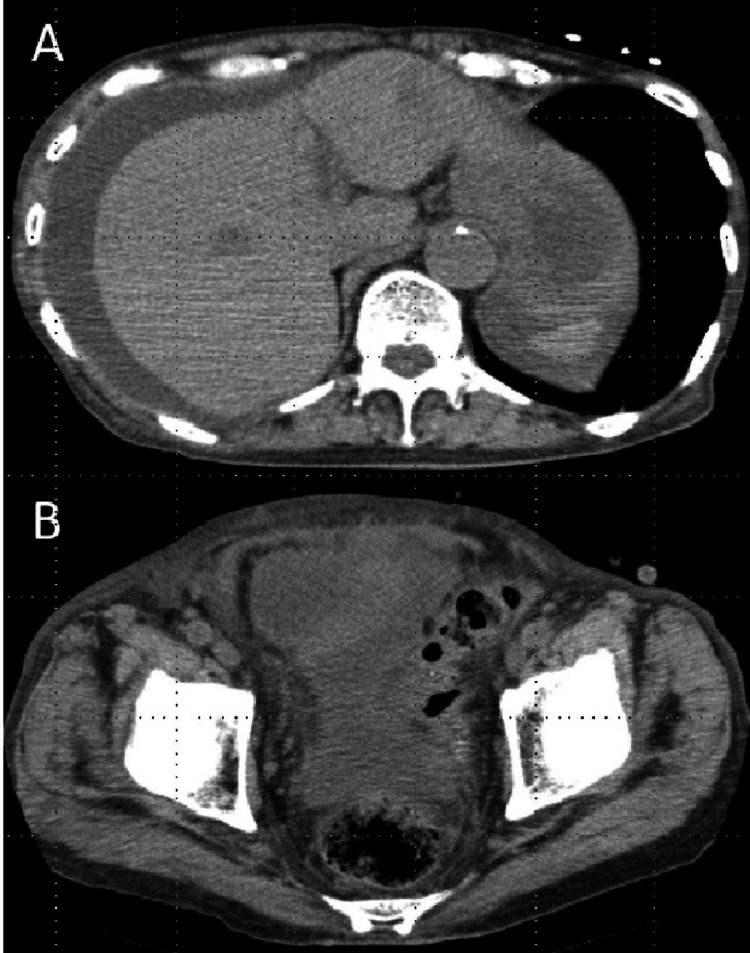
Abdominal computed tomography on admission Computed tomography revealed large amounts of hemorrhagic ascites in the abdominal cavity (A) and large amounts of ascites in the pelvic cavity (B).
